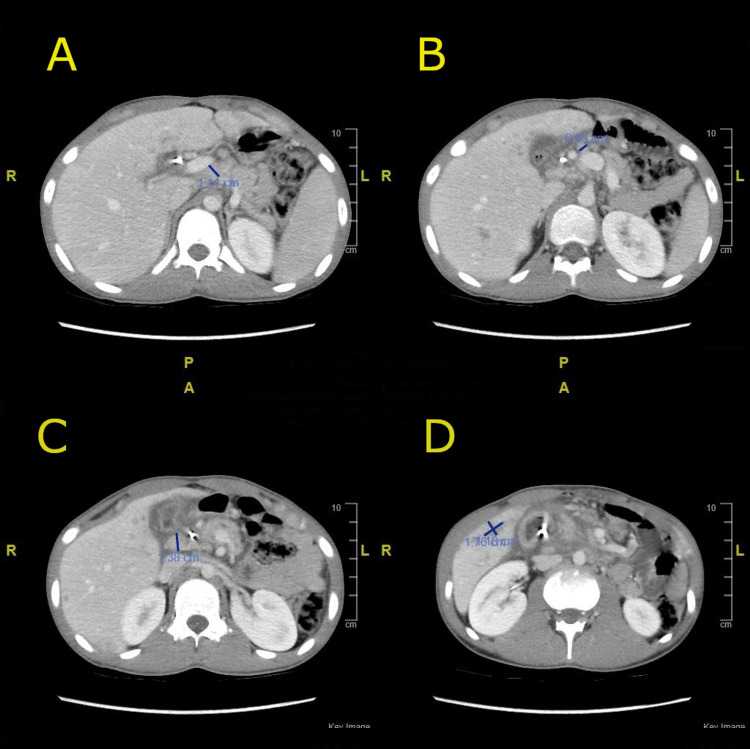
CT scan of the abdomen upon admission A: Axial CT image showing enlarged lymph node in the celiac region measuring 1.11 cm. B: Axial CT image showing enlarged lymph nodes in the para-aortic region measuring 0.63 cm. C: Axial CT image showing enlarged upper abdominal lymph nodes, the largest of which is in the porta hepatis, measuring 1.38 cm. D: Axial CT image showing multiple ill-defined focal hypodense hepatic lesions, the largest of which measures 1.8 × 1.2 cm. CT: computed tomography
